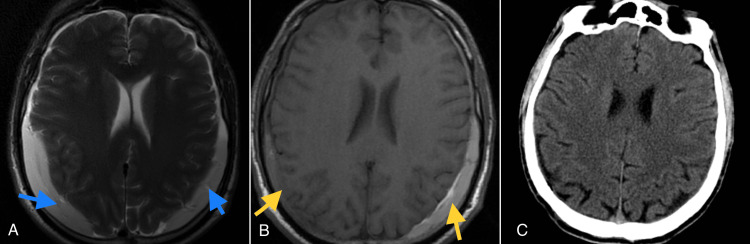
Evolution of a bilateral cSDH before and after MMAE. A: Cranial MRI showing bilateral cSDHs at presentation; B: Cranial MRI three weeks Post-MMAE; C: Cranial CT scan six weeks post-MMAE.
